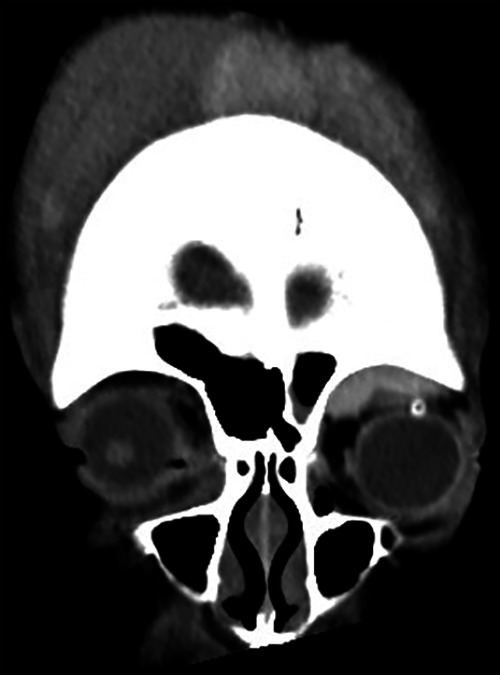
CT performed on the 5th day after surgery showed a large amount of subaponeurotic hemorrhage on the scalp, intraorbital hematoma was significantly reduced compared to the time of admission, and an inserted drain tube was observed.
